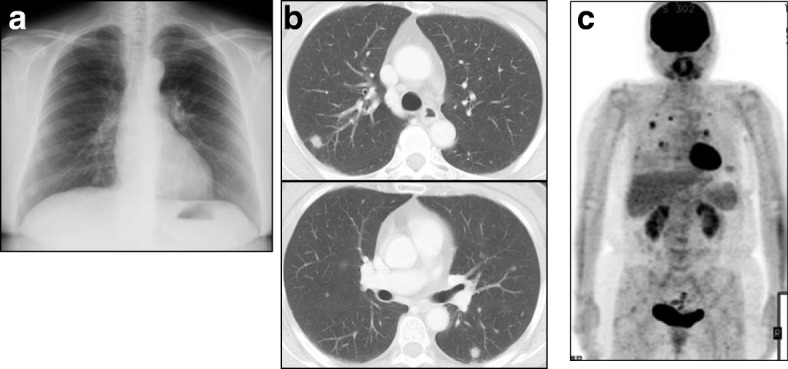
a, b Chest X ray (a) and chest computed tomography on admission (b) revealed multiple well-defined nodules in both lungs. c Fluorodeoxyglucose (FDG)-positron emission tomography revealed abnormal FDG uptake in the same lesions with a maximal standardized uptake value (SUV [max]) of 5.6
